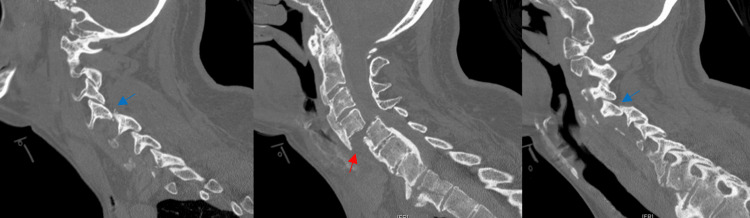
CT of the cervical spine on a patient with diffuse idiopathic skeletal hyperostosis from the data set demonstrating fracture/dislocation at the C4-5 level (center, red arrow), and bilateral perched facet joints (left, right panes, blue arrows).
